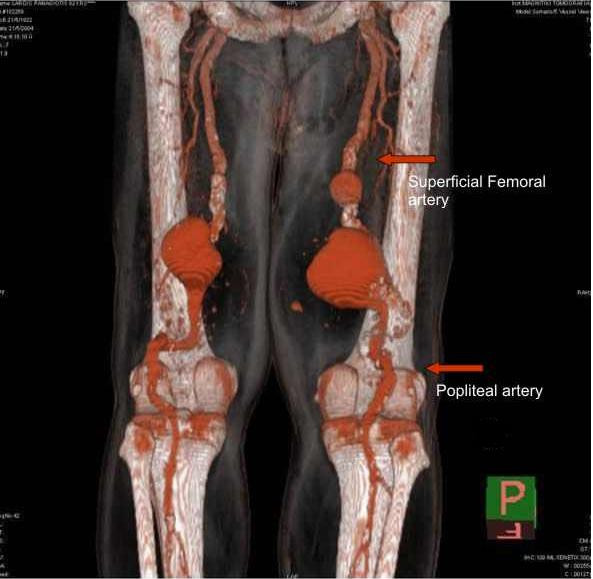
CT angiography results. A, B: 3D CT angiograms indicating the functional lumen of the two femoropopliteal aneurysms. C: CT scan indicating the maximum diameters of the popliteal aneurysms (10.5 cm on the right, 8.5 cm on the left).
